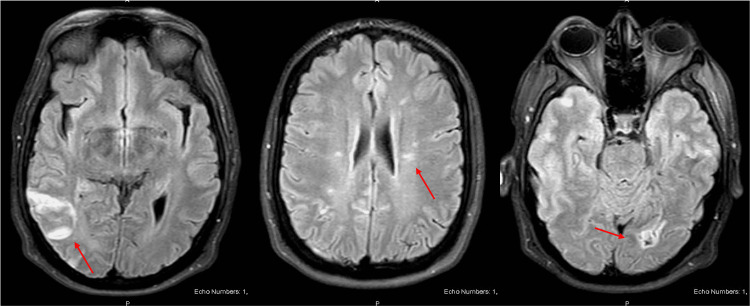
Non-contrast brain MRI images showing evidence of strokes (red arrow) in the right middle cerebral artery territory (left image), left cerebral hemisphere (middle image), and left posterior cerebral artery territory (right image). MRI: magnetic resonance imaging
